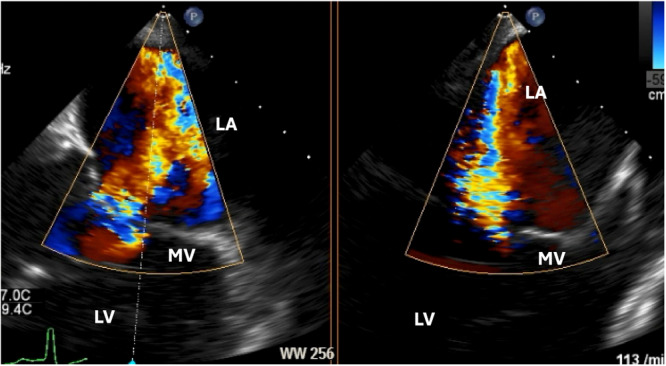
Baseline transesophageal echocardiography. Figure 1 shows transesophageal imaging of severe secondary ventricular mitral regurgitation. Imaging quality was impaired due to artefacts of the implanted Impella CP pump. [Color figure can be viewed at wileyonlinelibrary.com]
